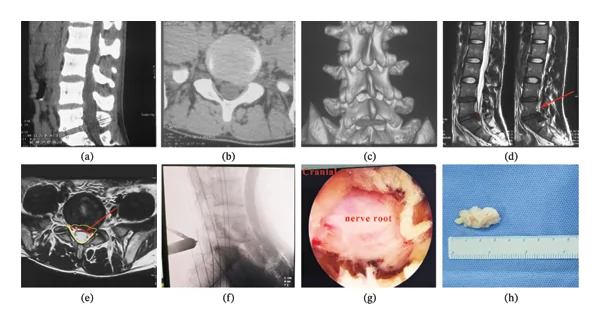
Preoperative multimodal imaging workup and intraoperative UBE decompression findings. (a–c) Preoperative lumbar computed tomography (CT) including (a) sagittal view, (b) axial view, and (c) 3D reconstruction showing intact bony alignment. (d, e) Preoperative lumbar magnetic resonance imaging (MRI) sequences. (d) Sagittal T2‐weighted images; the bidirectional red arrow (left panel) delineates the vertical craniocaudal extent of the focal canal stenosis, and the single red arrow (right panel) indicates the redundant nerve roots (RNRs). (e) Axial T2‐weighted view at the L5–S1 level; the yellow shaded region outlines the true cross‐sectional area of the spinal canal, whereas the area enclosed between the red curve and the anterior disc‐side yellow margin represents the relative intracanal space‐occupying disc mass. (f–h) Sequential intraoperative UBE landmarks: (f) fluoroscopic localization of the target level, (g) complete direct visualization of the thoroughly decompressed left S1 nerve roots, and (h) the final excised mass of the nucleus pulposus fragment.
